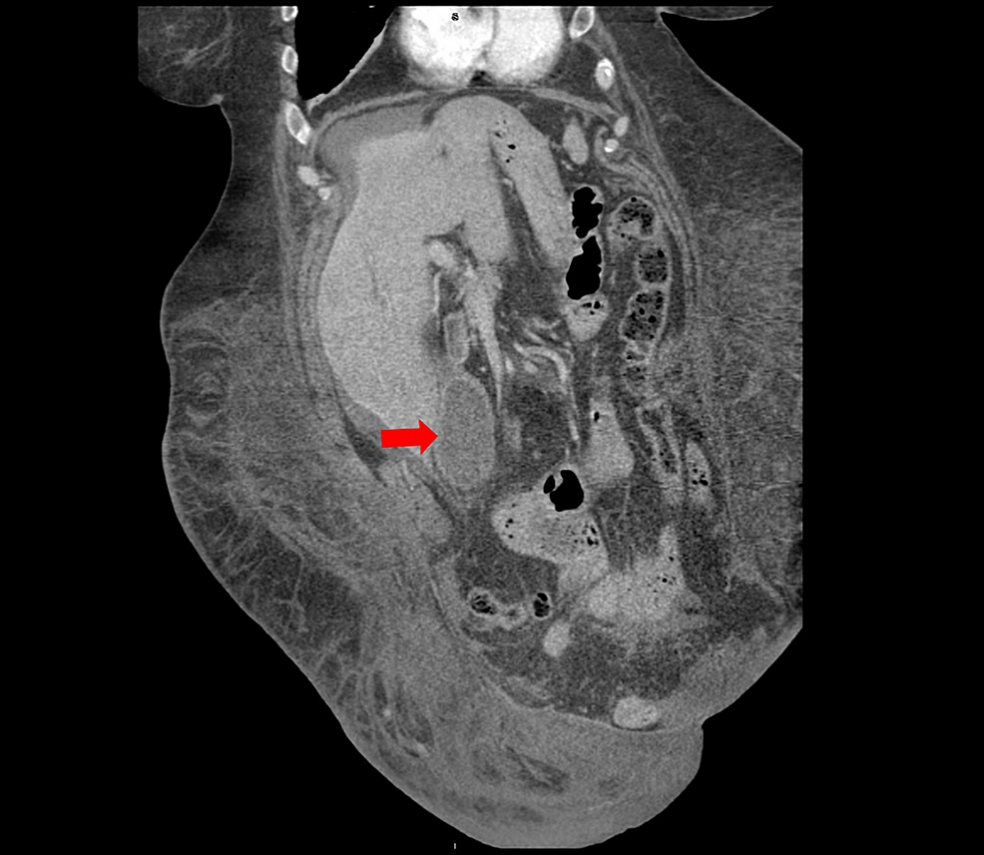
Coronal CT demonstrating the inferior location of the gallbladder. This figure demonstrates the anatomical position of the patient in the supine position. Note the inferior location of the gallbladder (red arrow) is noted to be in the right lower quadrant.
